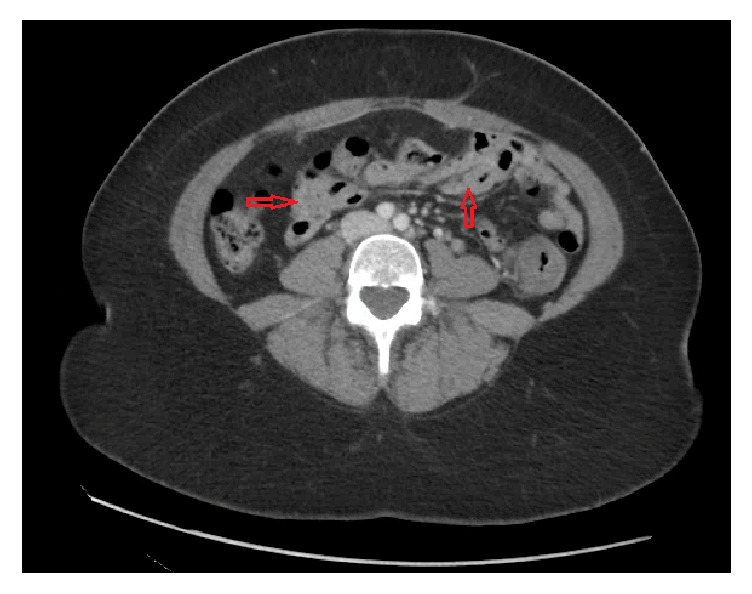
Transverse section of computed tomography scan demonstrating mild to moderate mural thickening of the descending/sigmoid colon (arrows) consistent with colitis without pericolonic abscess, ascites, or free air.
